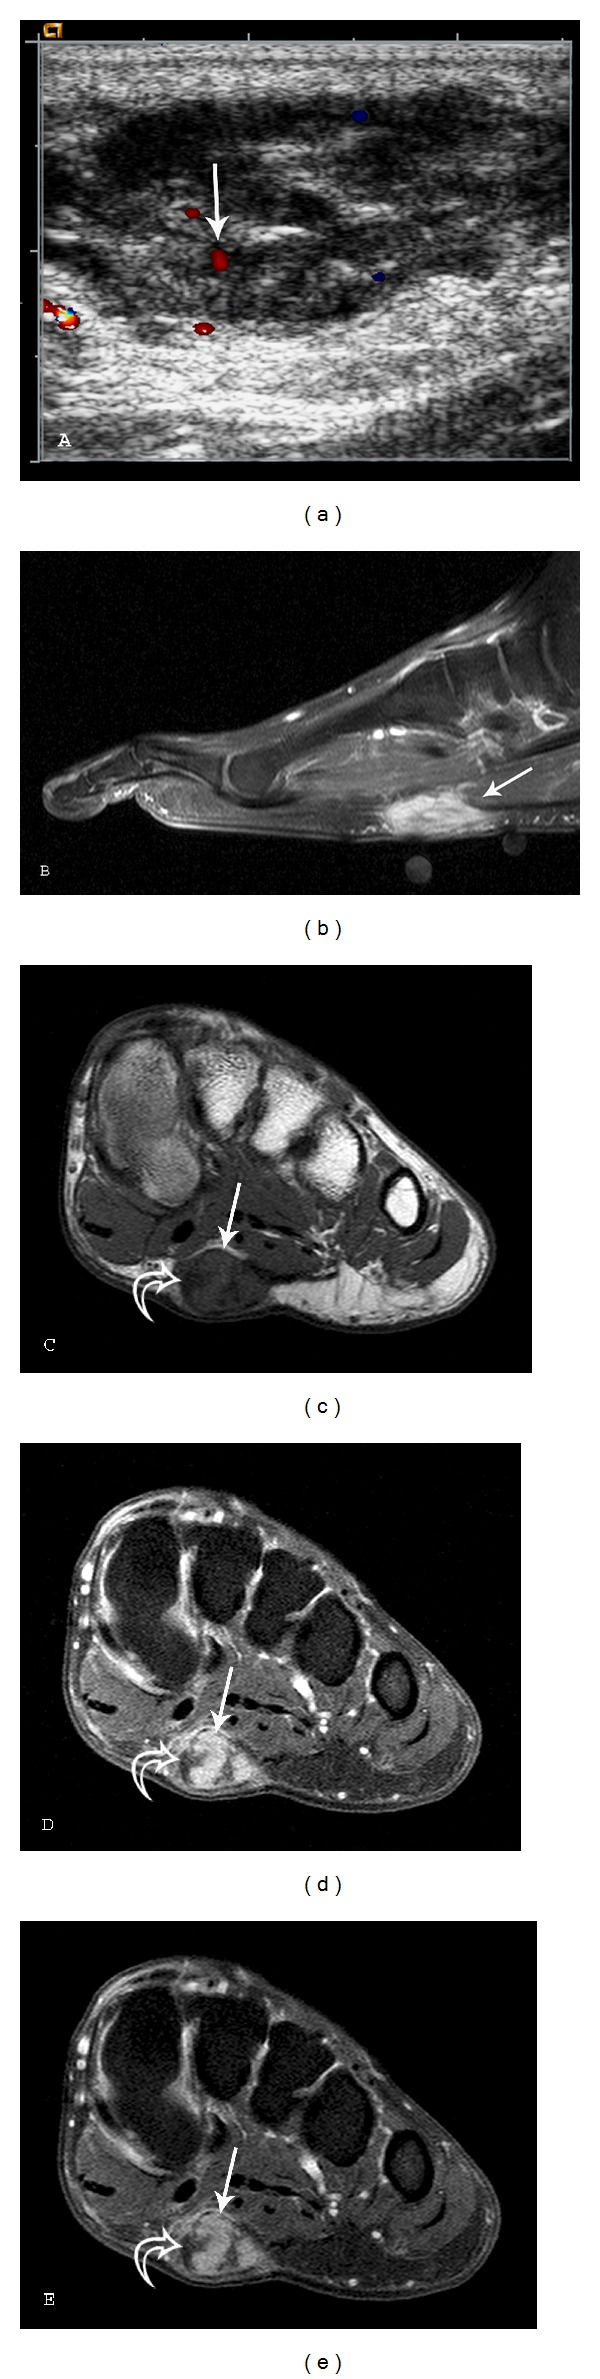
Plantar fibromatosis: a 54-year-old male who presents with left foot pain for one year. A longitudinal ultrasound color Doppler image (a) demonstrates a soft tissue mass with heterogeneous echotexture and internal color Doppler flow (arrow). (b) Sagittal T1-weighted (TR539.4/TE15) fat saturation postcontrast sequence demonstrates a fusiform, enhancing lesion with linear extension (fascial tail sign) along the plantar aponeurosis (arrow). Short axis MR images ((c)–(e)) demonstrate a well-defined mass (arrows) in the medial aspect of the plantar aponeurosis (c) Short axis T1-weighted (TR568/TE15) sequence reveals lesion signal intensity similar to skeletal muscle. There is heterogeneity with several foci of low signal (curved arrows) within the lesion. (d) Short axis T2-weighted (TR2693/TE60) with fat suppression reveals intermediate-to-high heterogeneous signal (arrow) and (e) T1-weighted (TR638.7/TE15) postcontrast fat saturation sequences demonstrate marked heterogeneous enhancement (arrow). Curved arrows indicate band-like areas of higher collagen content and low cellularity. Note the lower T1 and T2 signal intensity and lack of enhancement in these foci.
